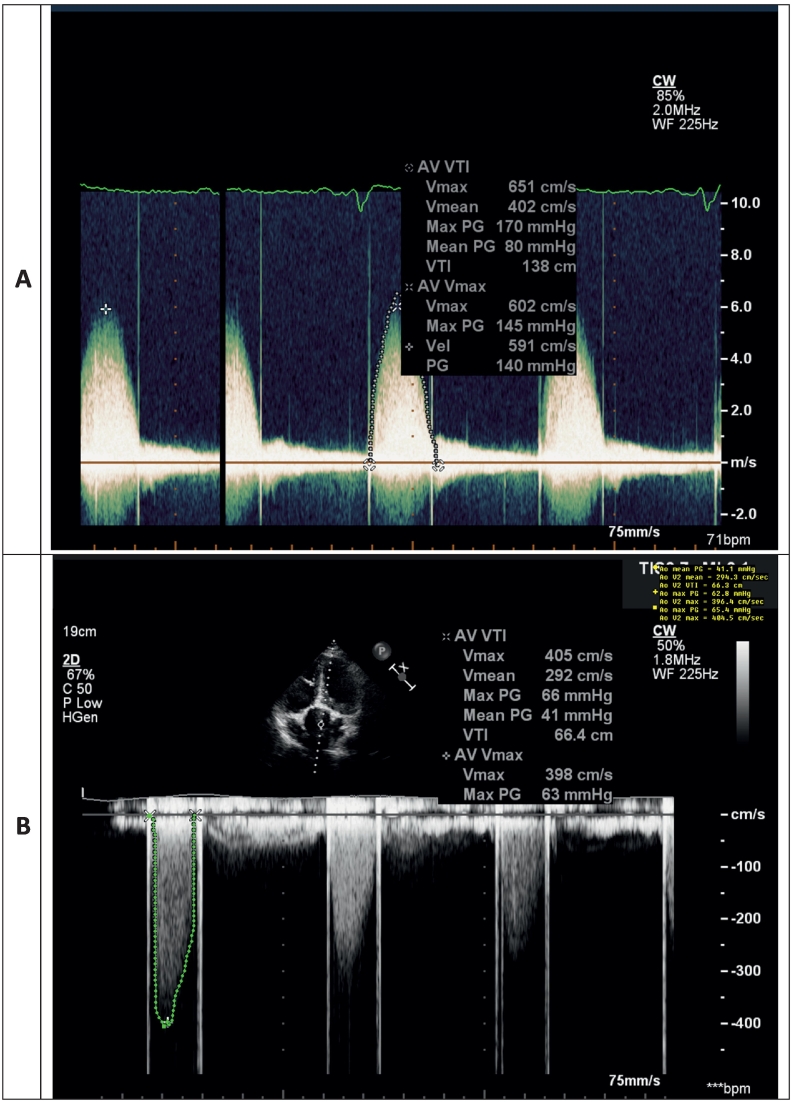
Echocardiography showed high peak and mean AV gradients (170 and 80 mm Hg, respectively) on the first day of admission (A) and reduced peak and mean AV gradients (66 and 41 mm Hg, respectively) on the 2nd day of admission (B).
